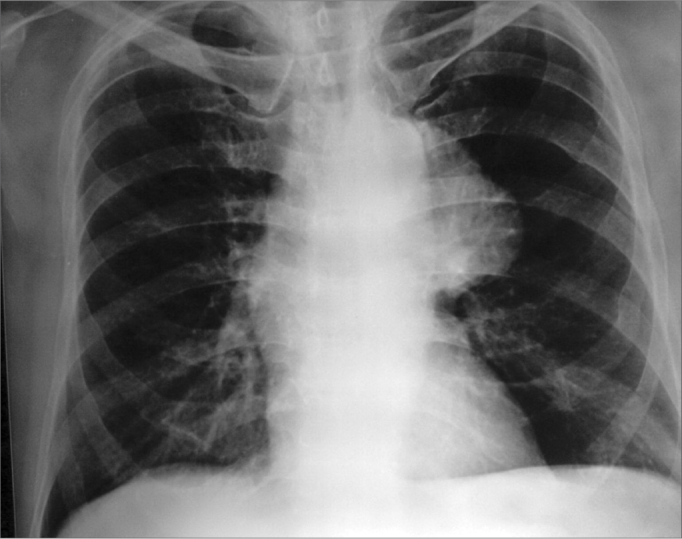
Chest X-ray PA view showing well- defined lobulated mediastinal lesion on the left side.
